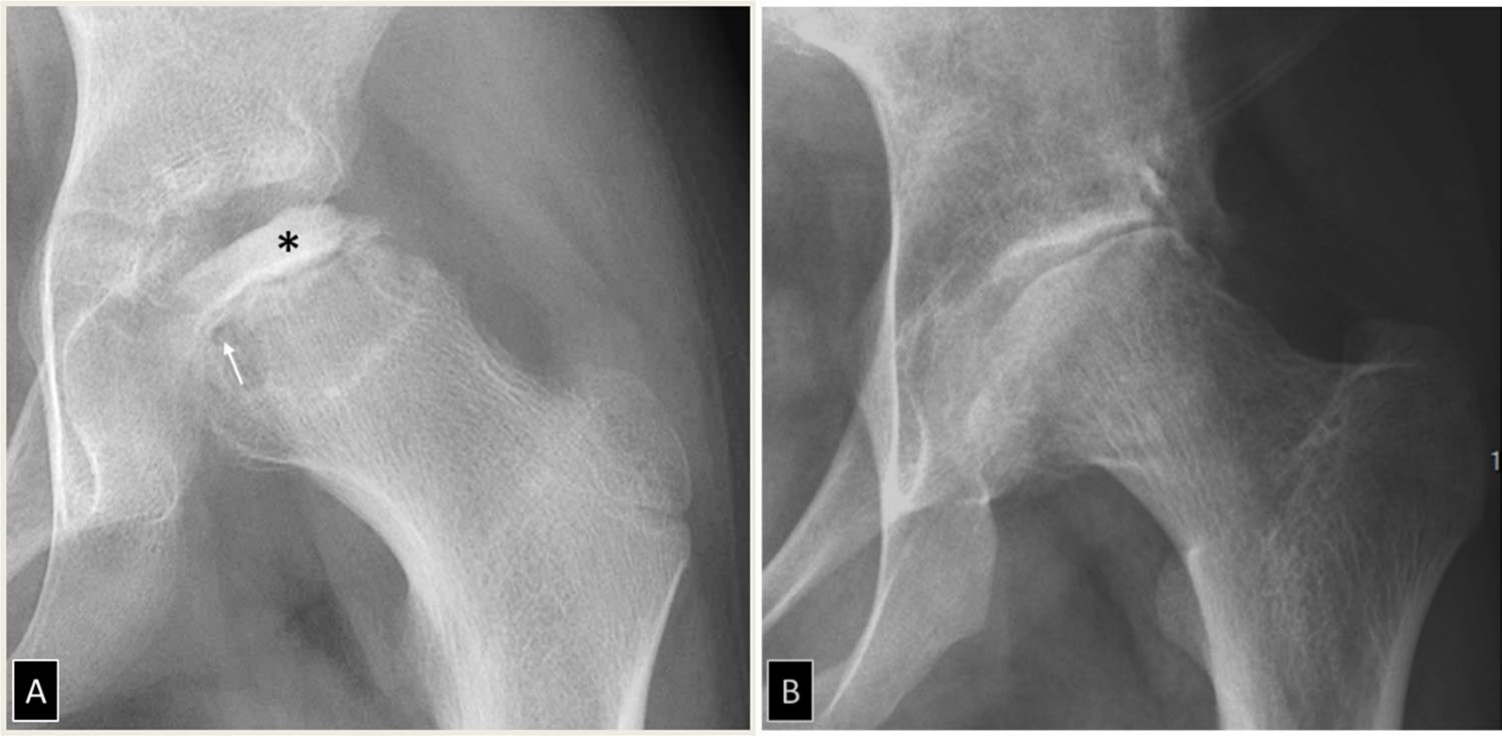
Secondary hip osteoarthritis. A AP radiograph of the left hip in an 8-year-old boy with Legg-Calvé-Perthes disease showing sclerosis of the femoral head epiphysis (asterisk) and cystic changes of the metaphysis (arrow). B Follow-up AP radiograph of the same patient at the age of 23 years showing secondary osteoarthritis with abnormal femoral head contours, articular incongruity, and joint space narrowing
